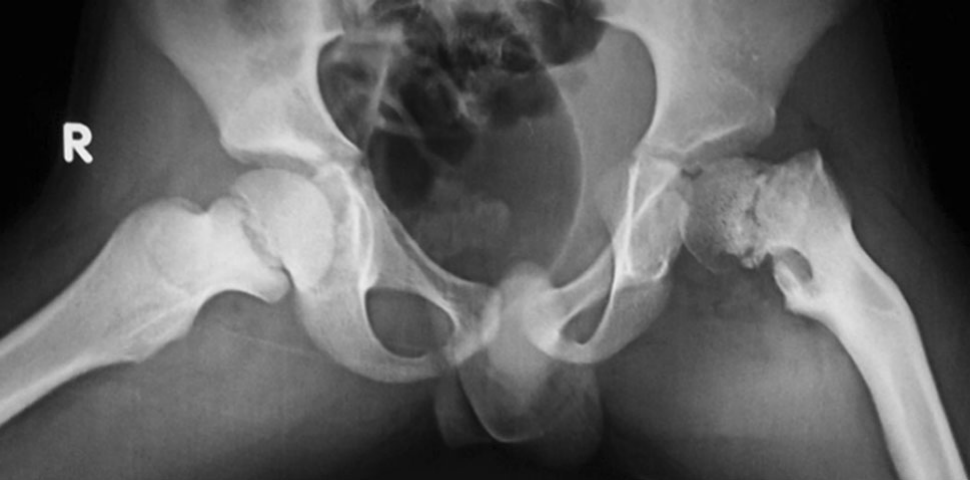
A standard frog-leg lateral radiograph of the pelvis and both hip joints showed a dysplastic proximal femur and acetabulum with a retroverted neck on the affected side
